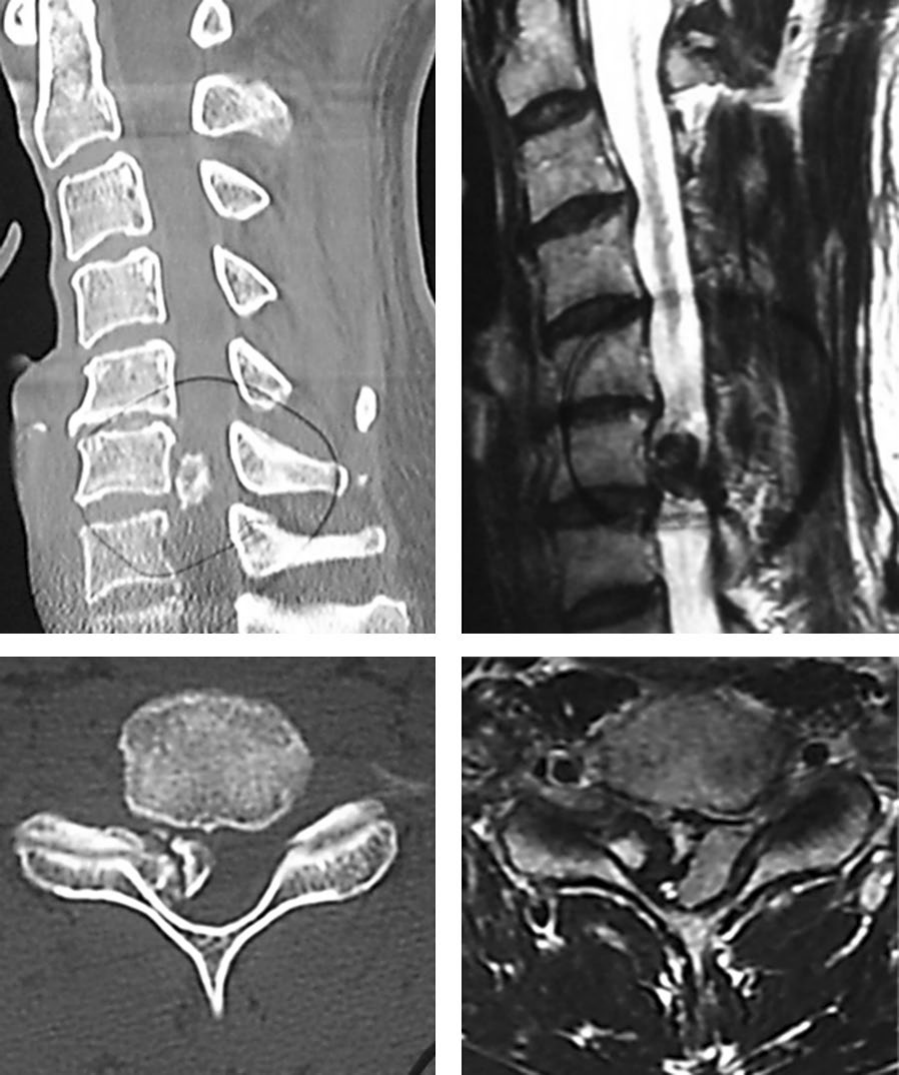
Preoperative CT and MRI of the cervical spine. Sagittal and axial CT scans demonstrated an osseous tumor originating in the neighborhood of the right C6/7 facet joint. Sagittal and axial MRI revealed an extradural mass which compressed the spinal cord severely at C6/7 level
